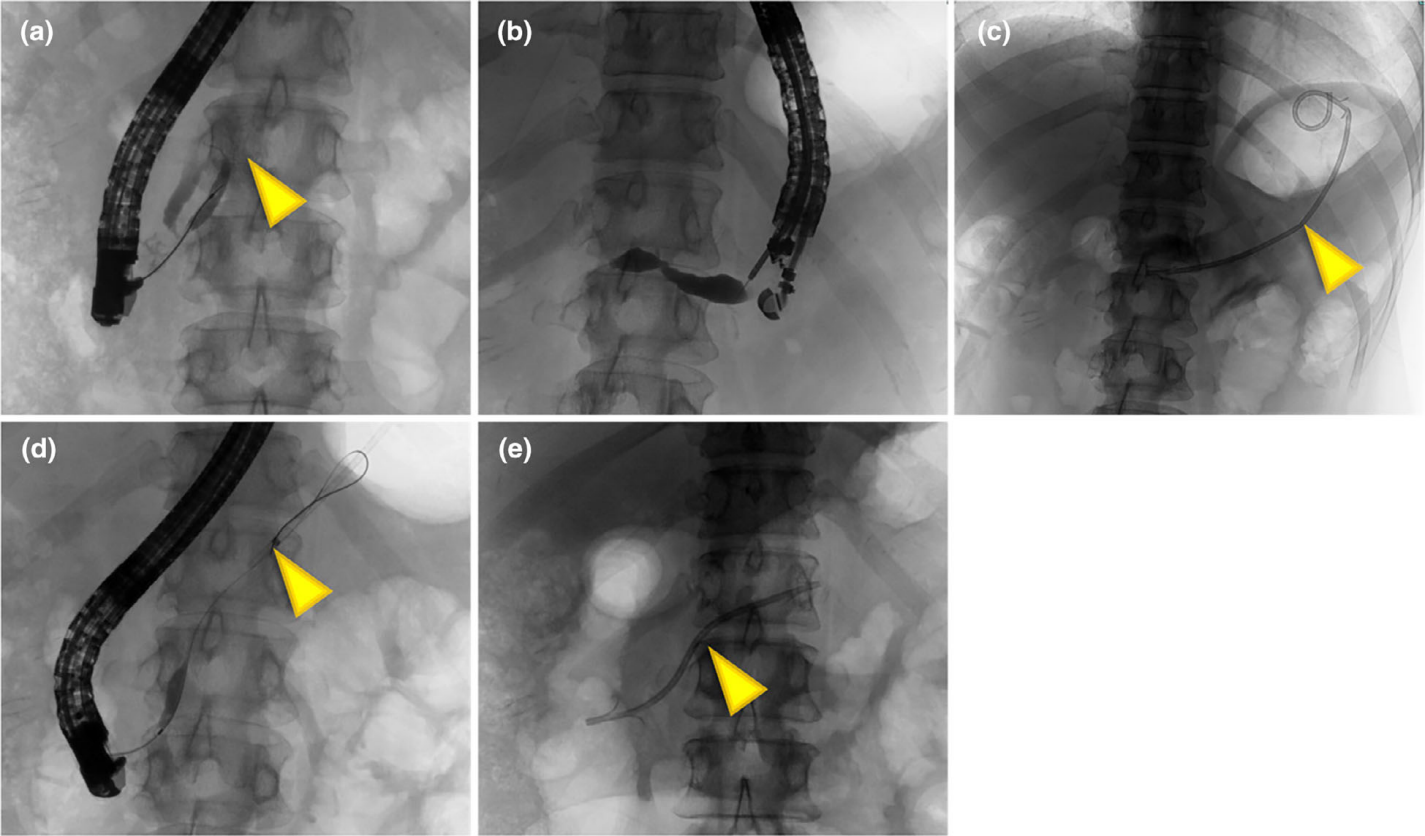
Endoscopic ultrasound‐guided pancreatic duct drainage. (a) Endoscopic retrograde pancreatography showing severe stricture (arrowhead). (b) A 19G needle was punctured into the main pancreatic duct (MPD), and a pancreatogram shows the dilated MPD. (c) Pancreaticogastrostomy was completed using a 7F plastic stent (PS) (arrowhead). (d) After 6 month, stricture (arrowhead) was possible to pass though by transpapillary approach. (e) 10F PS placement (arrowhead).
